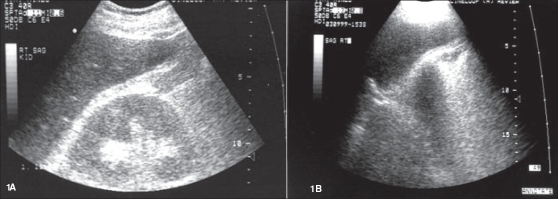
Two ultrasound images of the right kidney obtained two days apart are shown. A previously well-visualized right kidney depicted in Figure 1A is no longer visible in Figure 1B
